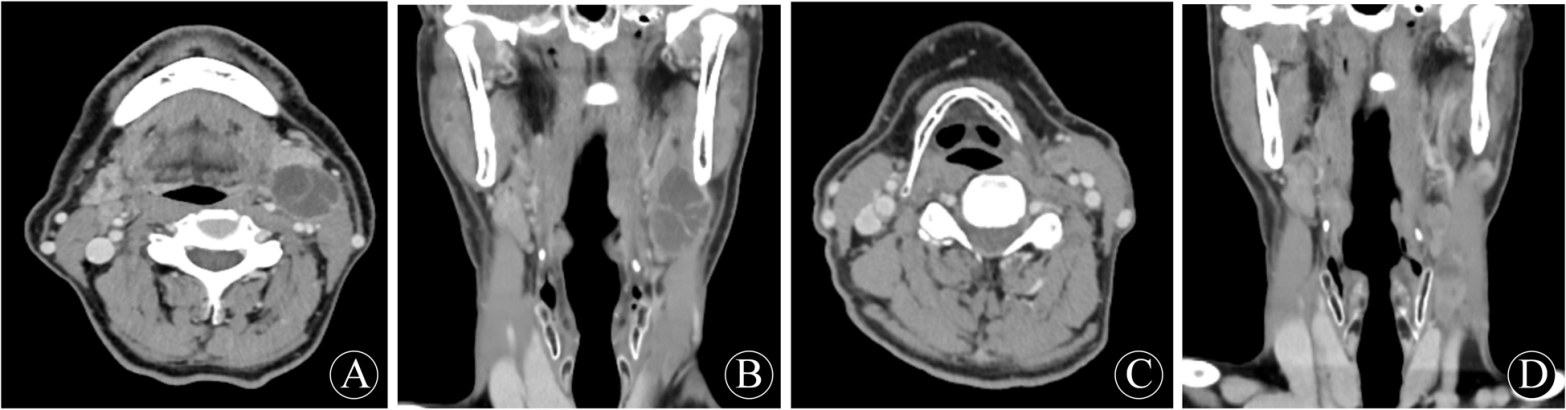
First preoperative neck enhancement CT performance: (A) transverse position; (B) coronal position. Second preoperative neck enhancement CT performance: (C) transverse position; (D) coronal position.
